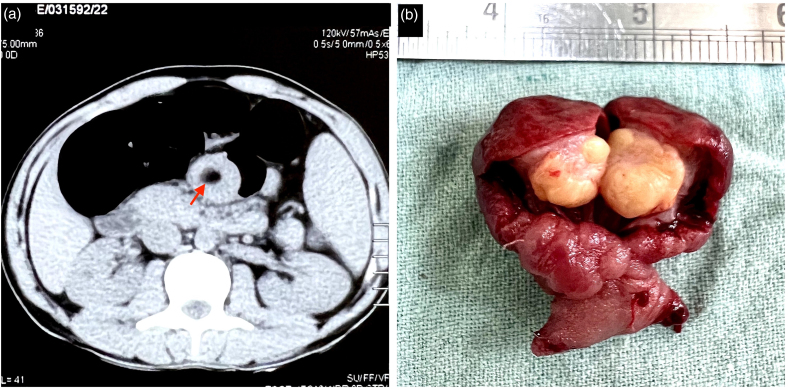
(a) Axial section of abdominal CT scan showing an adipose laden lesion (arrow) in the jejunum. (b) Gross examination of the resected jejunal segment showing a lipomatous lesion.
